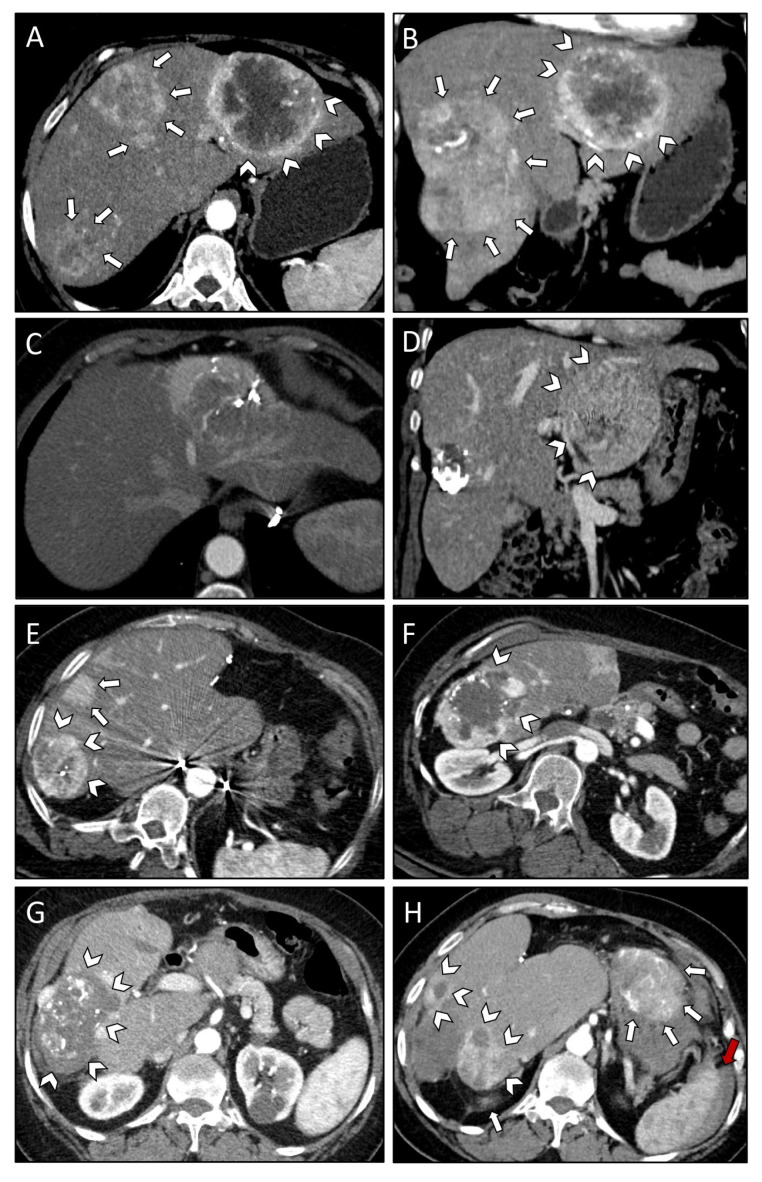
Axial (A) and coronal plane (B) of initial CT scans demonstrating multiple large SFT/HPC liver metastases affecting both right (white arrows) and left (white arrowheads) liver lobe. Axial (C) and coronal (D) plane of control CT scans following a total of six TAE sessions revealing mixed response with residual vital tumor tissue in liver segment II/III (white arrowheads; (D)). CT scans nine months after resection of left lateral segments revealing recurrent lesions in segments V, VI, VII (white arrowheads; (E,F)) and two new lesions in segments IVa and VII (white arrows; (E)). Successful treatment of recurrent lesions after a total of six SRFA and one additional TAE session as shown in the first follow-up CT scan after the last SRFA (white arrowheads; (G)). CT scan obtained following syncope 18 months after the last intervention revealing diffuse metastatic liver disease (white arrowheads; (H)), peritoneal metastases (white arrows; (H)), and ascites (red arrow; (H)) 18 months after the last intervention.
